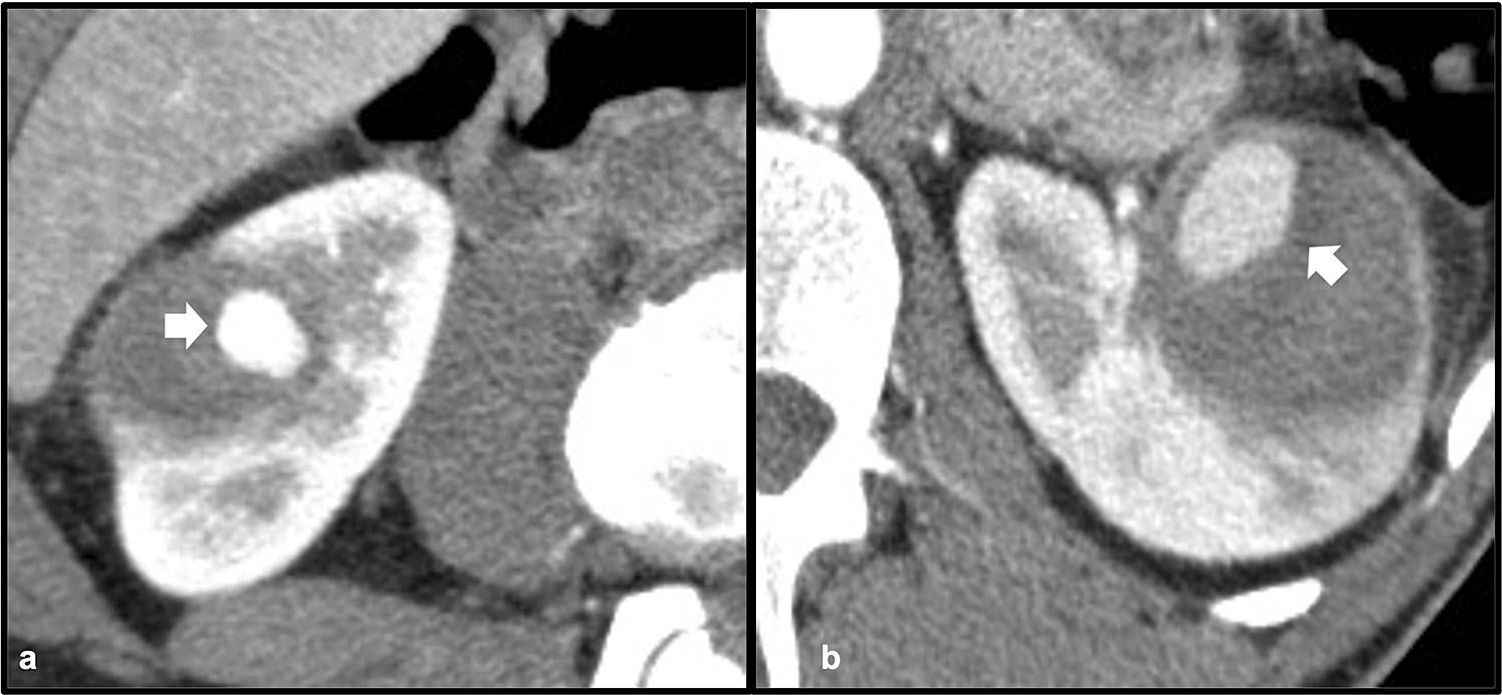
48-year-old male with a history of intravenous drug abuse who presented with flank pain and hematuria 3 weeks following coronary angiography and stenting. Axial post-contrast CT (a-b) demonstrates bilateral renal artery aneurysms (white arrows) consistent with intraparenchymal mycotic aneurysms. These were treated with embolization
